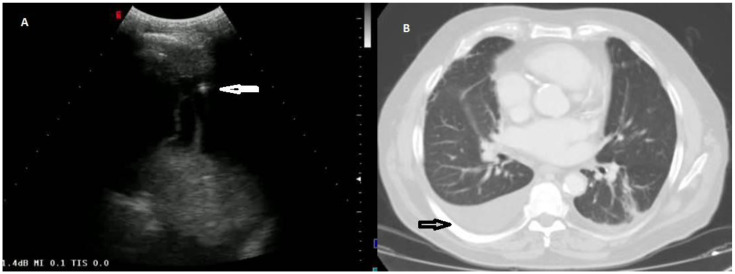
(A) TUS scan showing an anechoic effusion with consensual parenchymal atelectasis during thoracentesis with a multifrequency convex probe (3.5 MHz). The tip of the needle is highlighted by a white arrow. (B) The corresponding CT scan shows a smooth thickening of the peribronchovascular interstitium and a bilateral pleural effusion with passive atelectasis of lower lobe in the right lung (black arrow).
